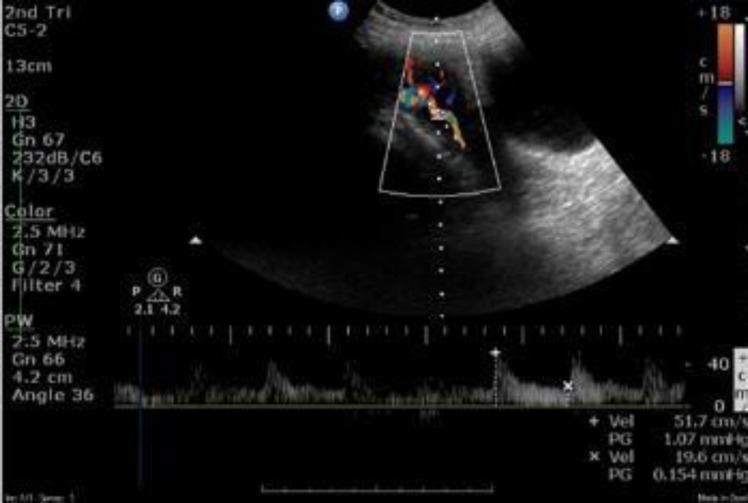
Doppler waveform of the uterine artery in the second trimester
